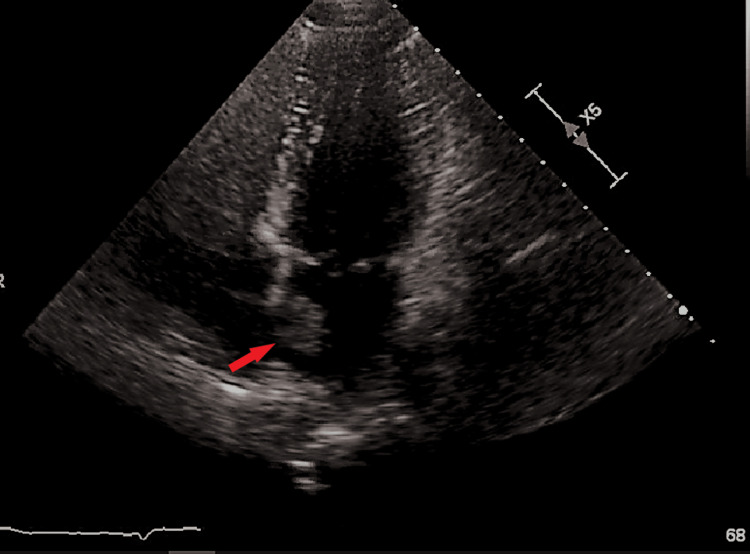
TEE, apical four-chamber view. The red arrow demonstrates a mobile mass on the left atrium, approximately 13 x 15 mm, with mild aortic insufficiency
